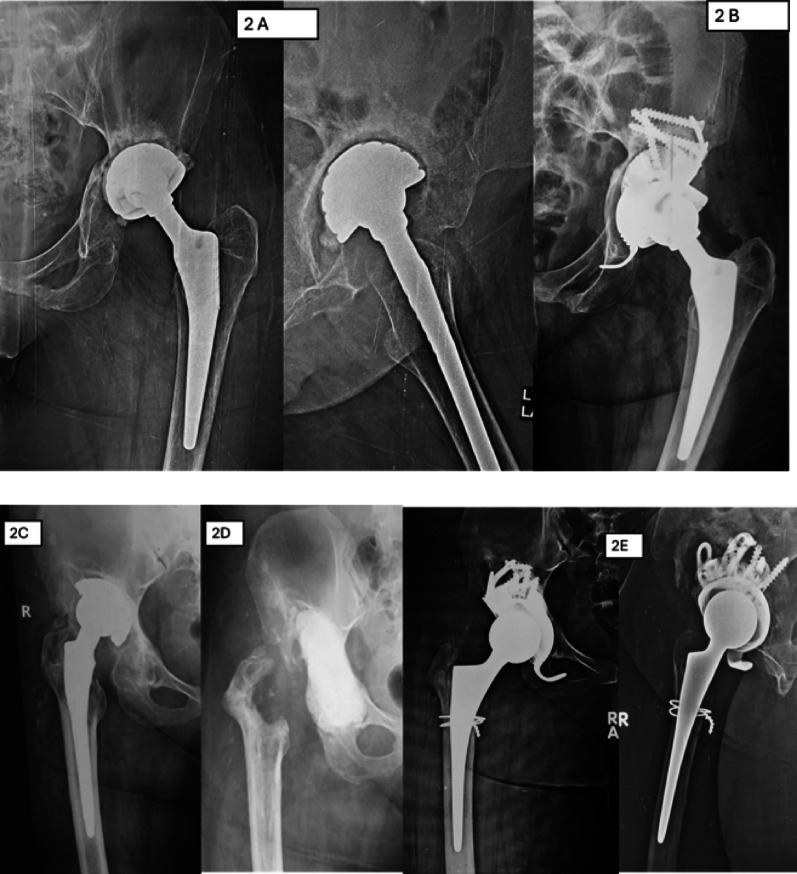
Case examples of Type III Paparosky management: A Preoperative radiograph showing failed hemiarthroplasty. B Postoperative radiograph at the final follow up visit after Delta TT system implantation. C Preoperative radiograph showing septic loosening of cementless acetabular cup. D Preoperative radiograph showing implant removal. E Postoperative radiograph at the final follow up visit after Delta TT system implantation
